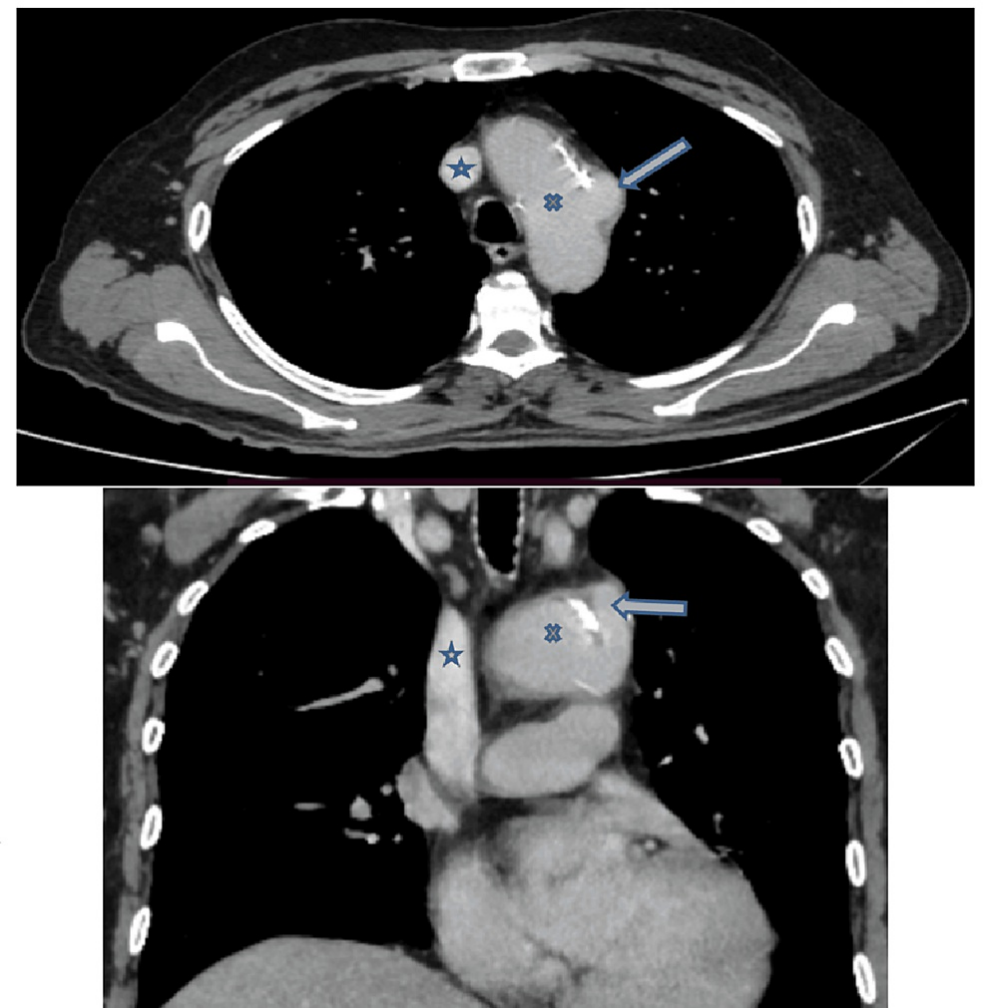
Computed tomography demonstrating the pseudoaneurysm of the aortic arch along with atherosclerotic changes. Pseudoaneurysm (arrow), aortic arch (cross), and superior vena cava (star).
